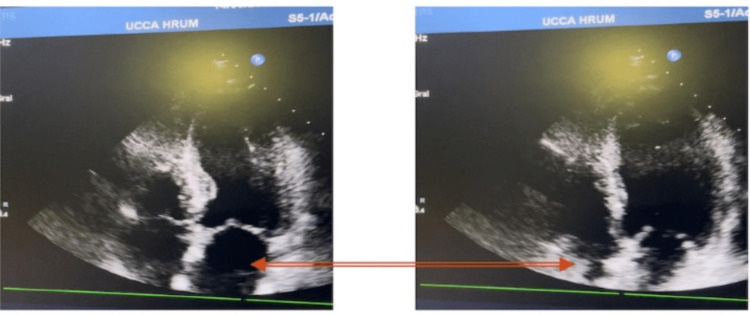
Post-myocardial reperfusion therapy echocardiography Post-myocardial reperfusion images (diastole (left) and systole (right)) show recovery of both left and right chambers. The ejection fraction was higher than 45%.
